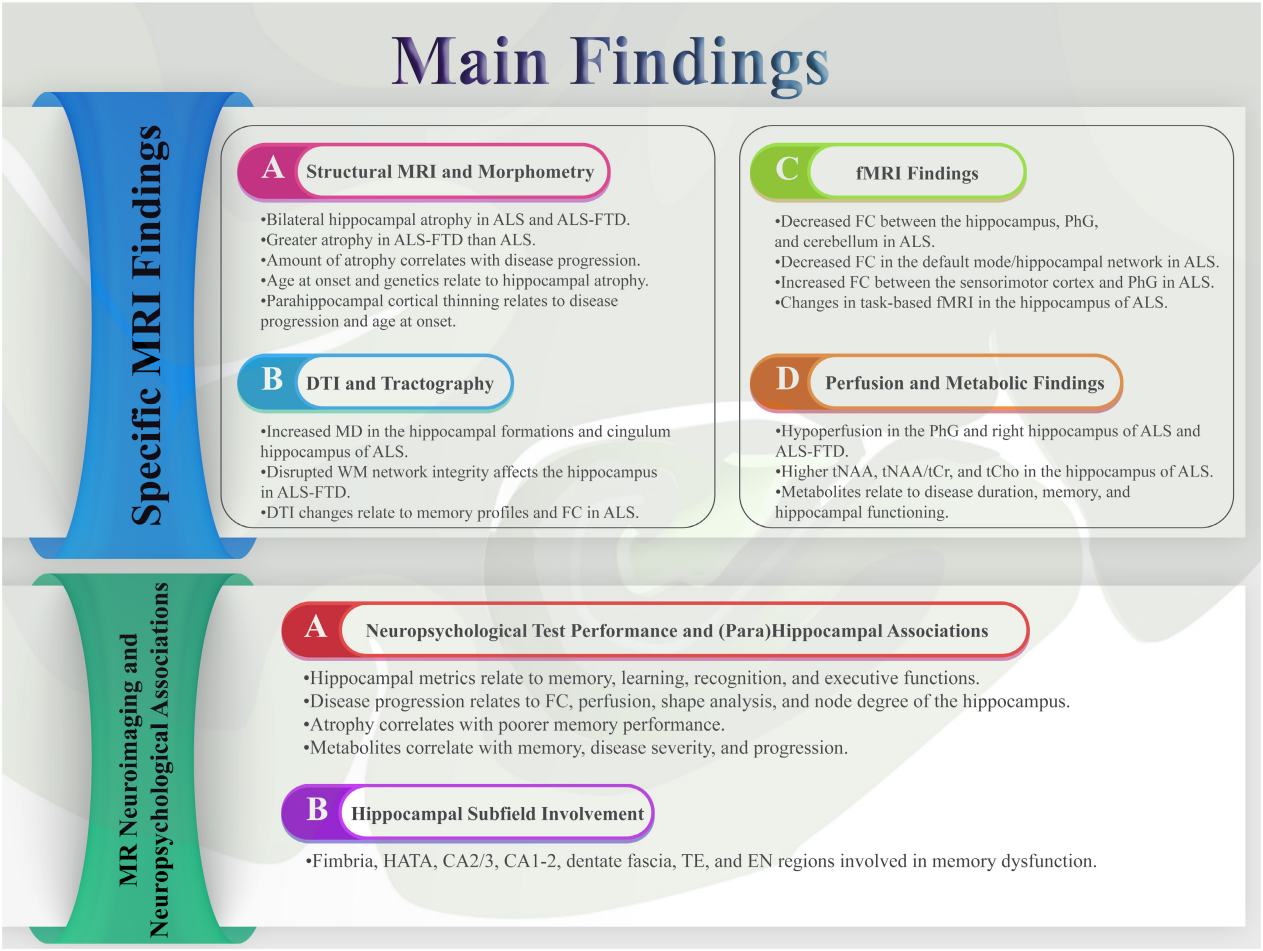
Primary MRI and neuropsychological findings of hippocampal and parahippocampal regions in ALS patients.
